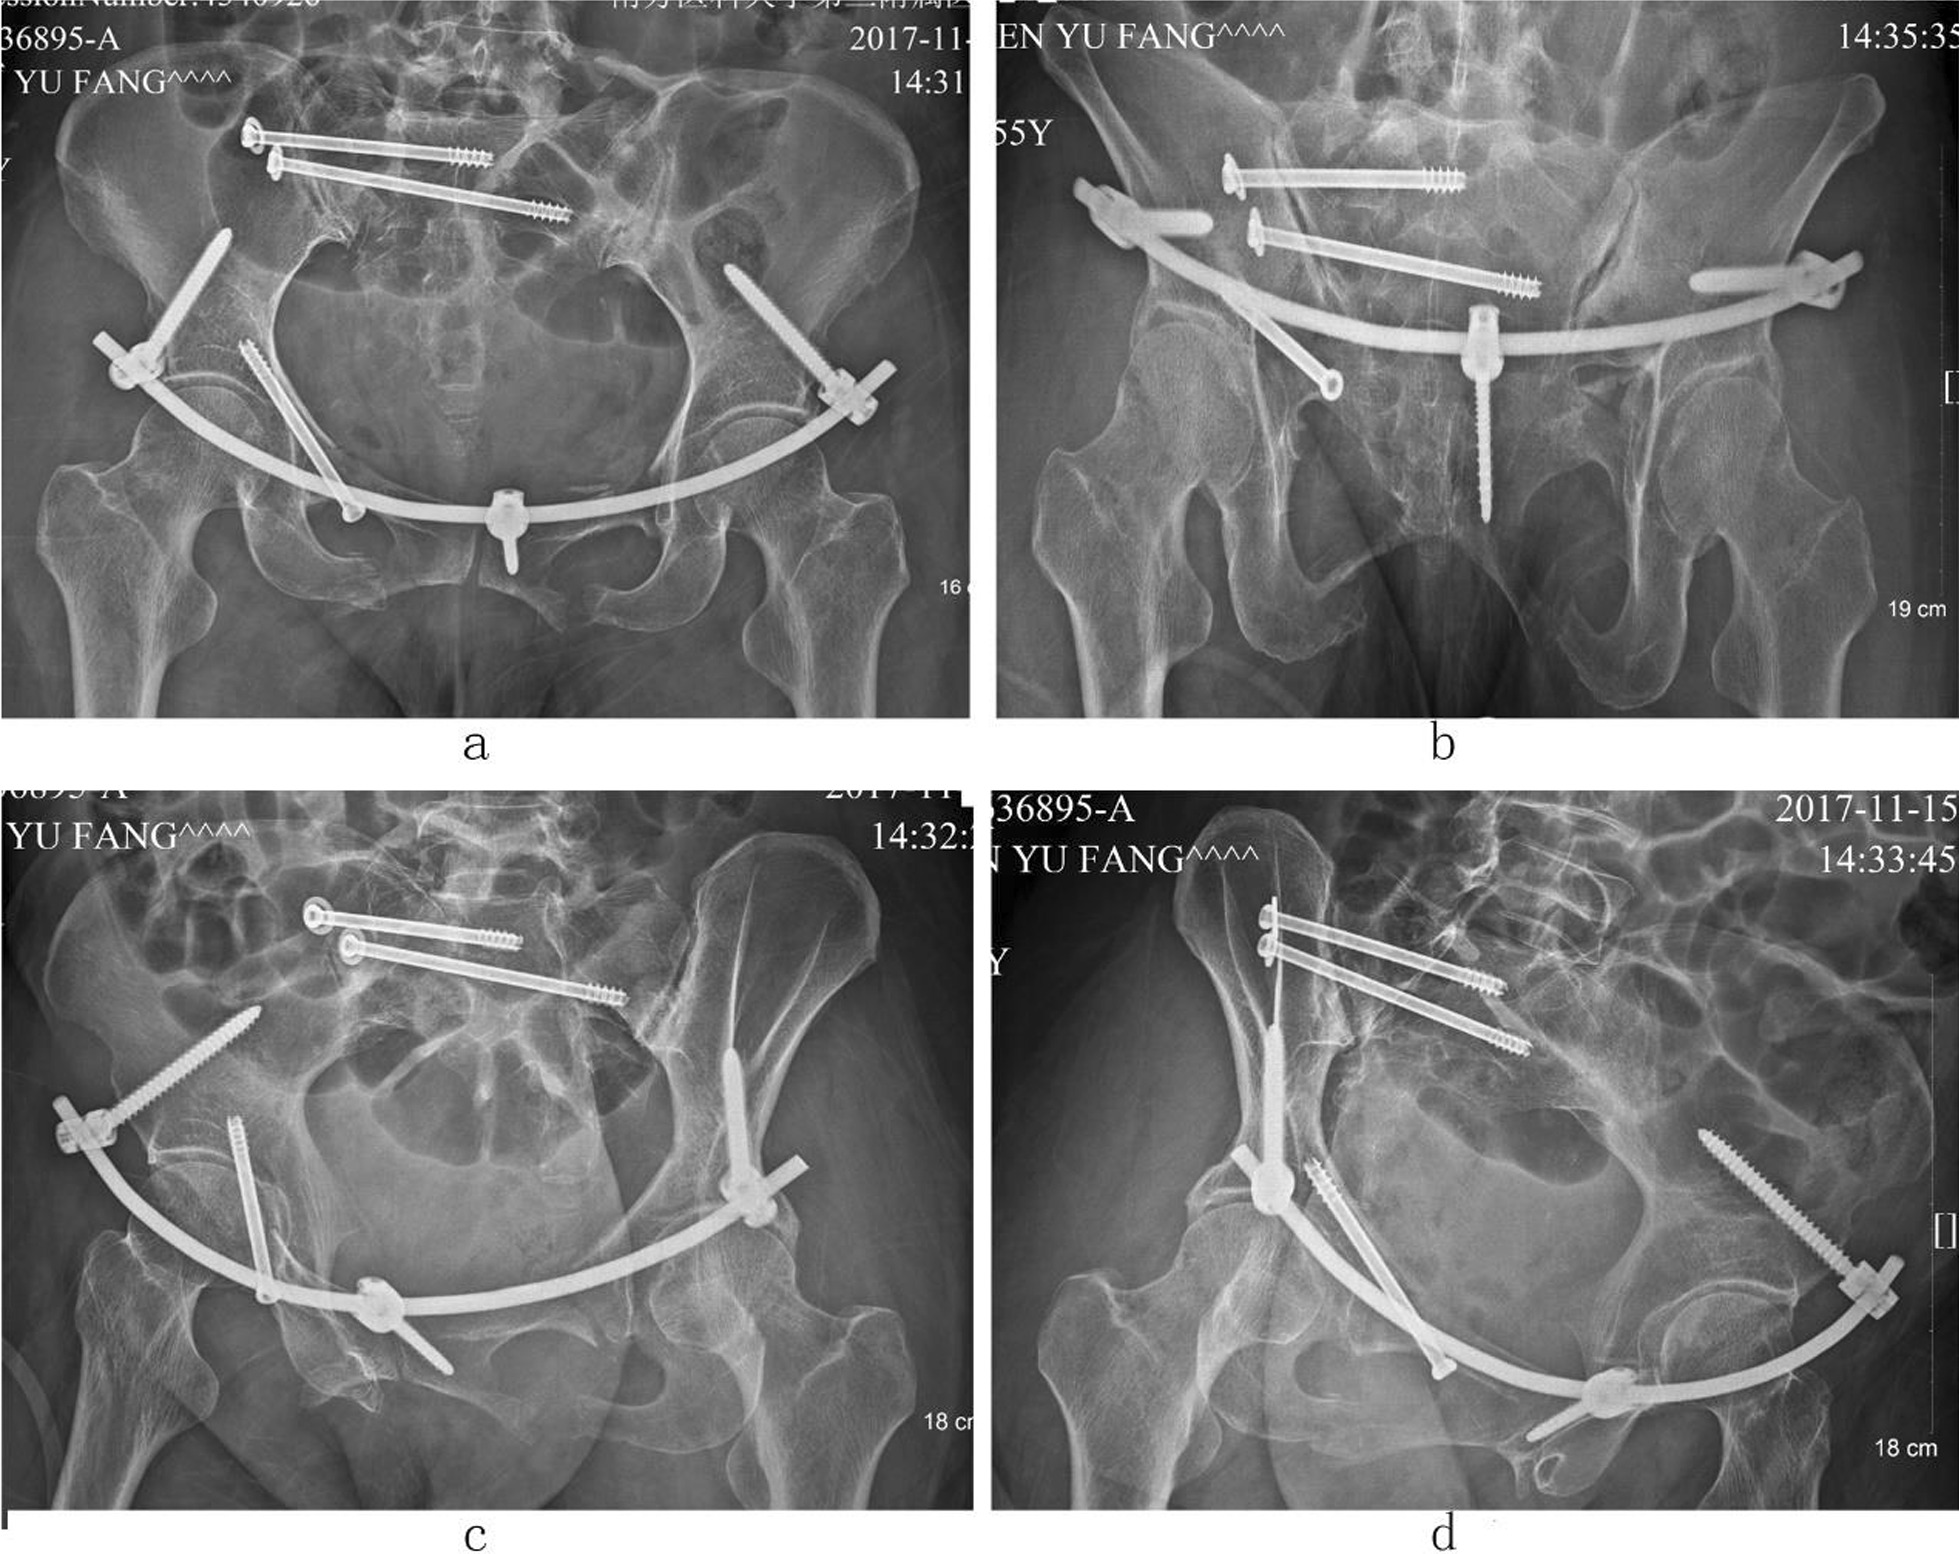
Postoperative X-ray images of case 2. (a Pelvic anteroposterior X-ray image, b pelvic outlet X-ray image, c iliac oblique position X-ray image, d obturator oblique position X-ray image.)
